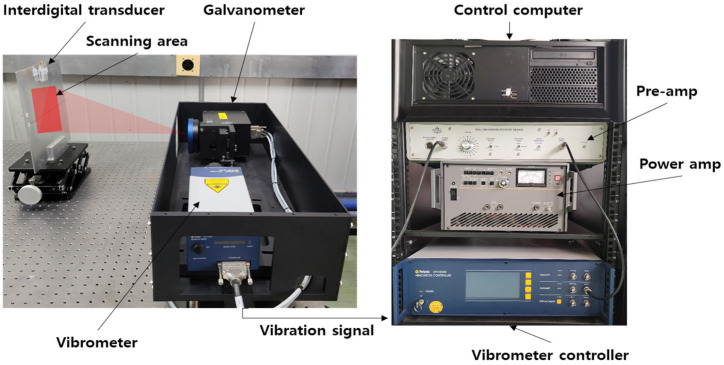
Setup for scanning laser Doppler vibrometer (SLDV) experiments.
